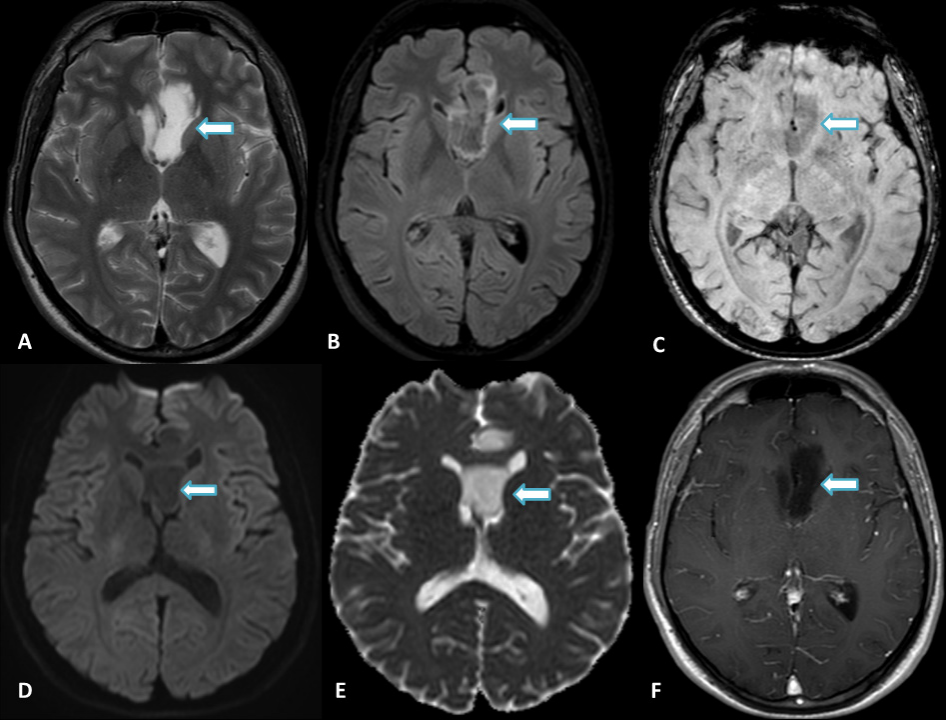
Male, 22 years old, presenting with progressive episodes of loss of consciousness, disconnected speech, and upper limb disorders started 7 months ago. Axial conventional MRI images demonstrate a large infiltrative lesion (arrows) in the left septal area (subcallosal gyrus) with a prominent hyperintense signal on T2WI (A), partially suppressed on FLAIR (B). Note also the well-defined hyperintense rim on FLAIR (B). SWI demonstrates low signal inside the lesion (C) and facilitated diffusion on DWI and ADC map, consistent with low cellularity (D and E). No contrast enhancement is seen (F).
